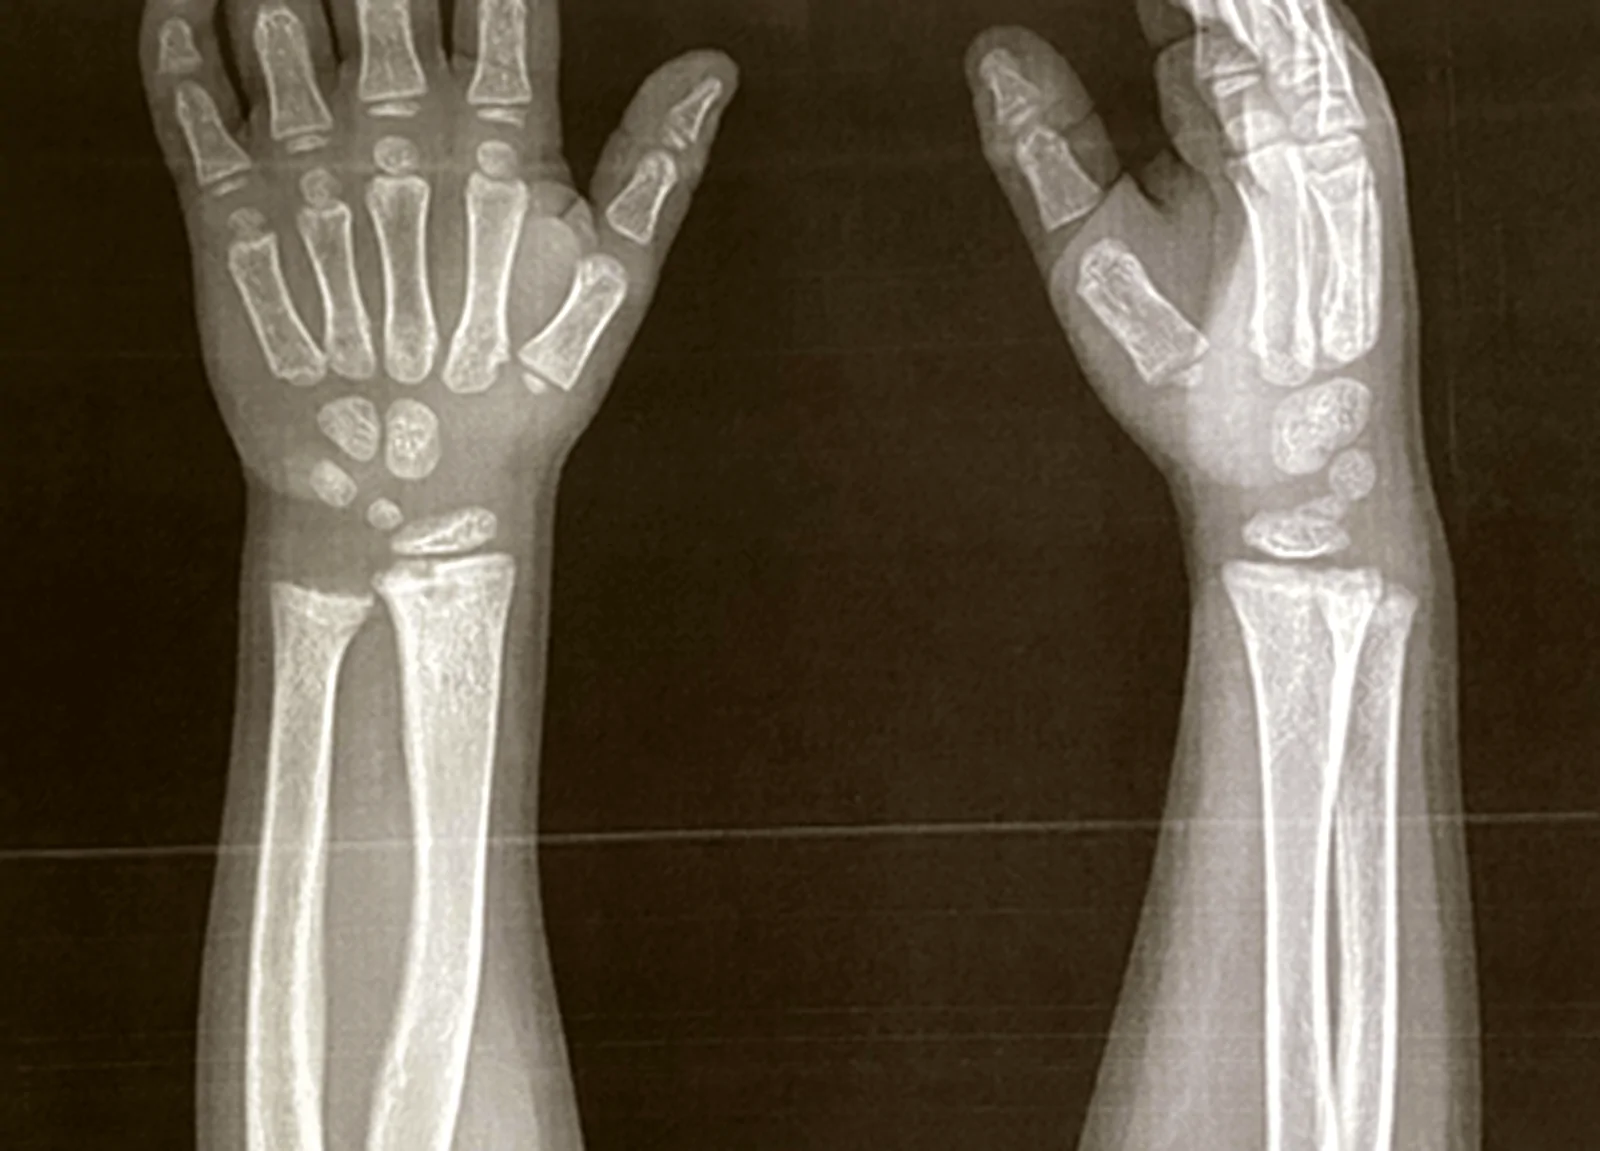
Wrist radiograph Anteroposterior and lateral radiographs of the wrist demonstrating distal metaphyseal widening of the radius and ulna, with fraying, characteristic findings of active rickets. No significant delay in bone age is observed
